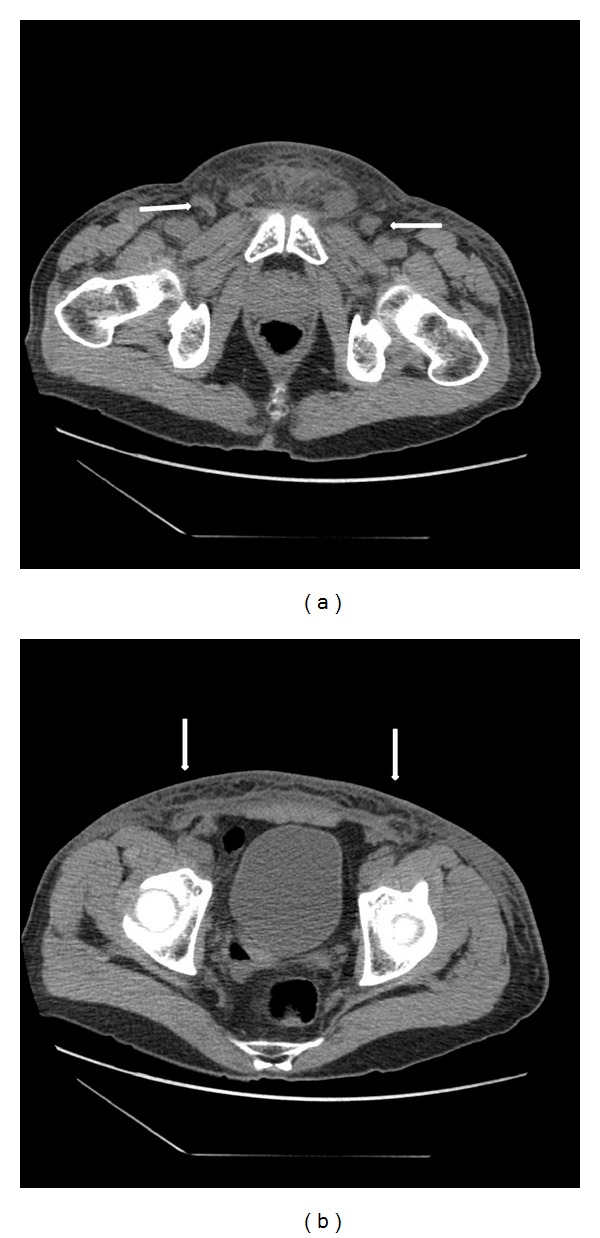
Computed tomography of abdomen and pelvis showing (a) extensive edema and stranding of subcutaneous tissue at the symphysis pubis and bilateral groin areas; bilateral enlargement of inguinal lymph nodes is also noted (two arrows). (b) Edema and fat stranding extending into subcutaneous tissue at anterior lower abdominal wall area (two arrows).
